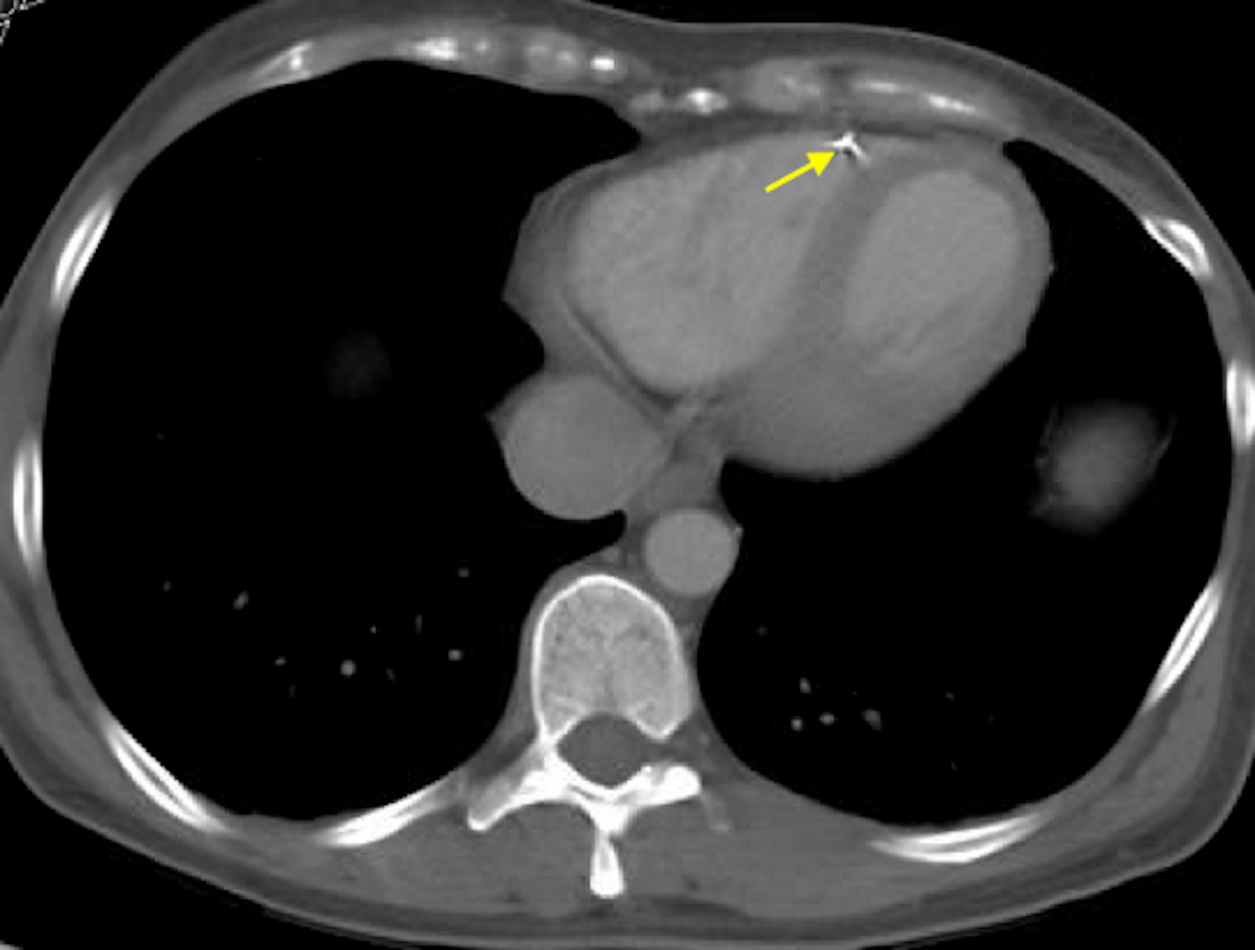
CT of the chest (axial section) showing one of the inferior vena cava filter struts embedded in the anterior wall of the right ventricle (yellow arrow).
